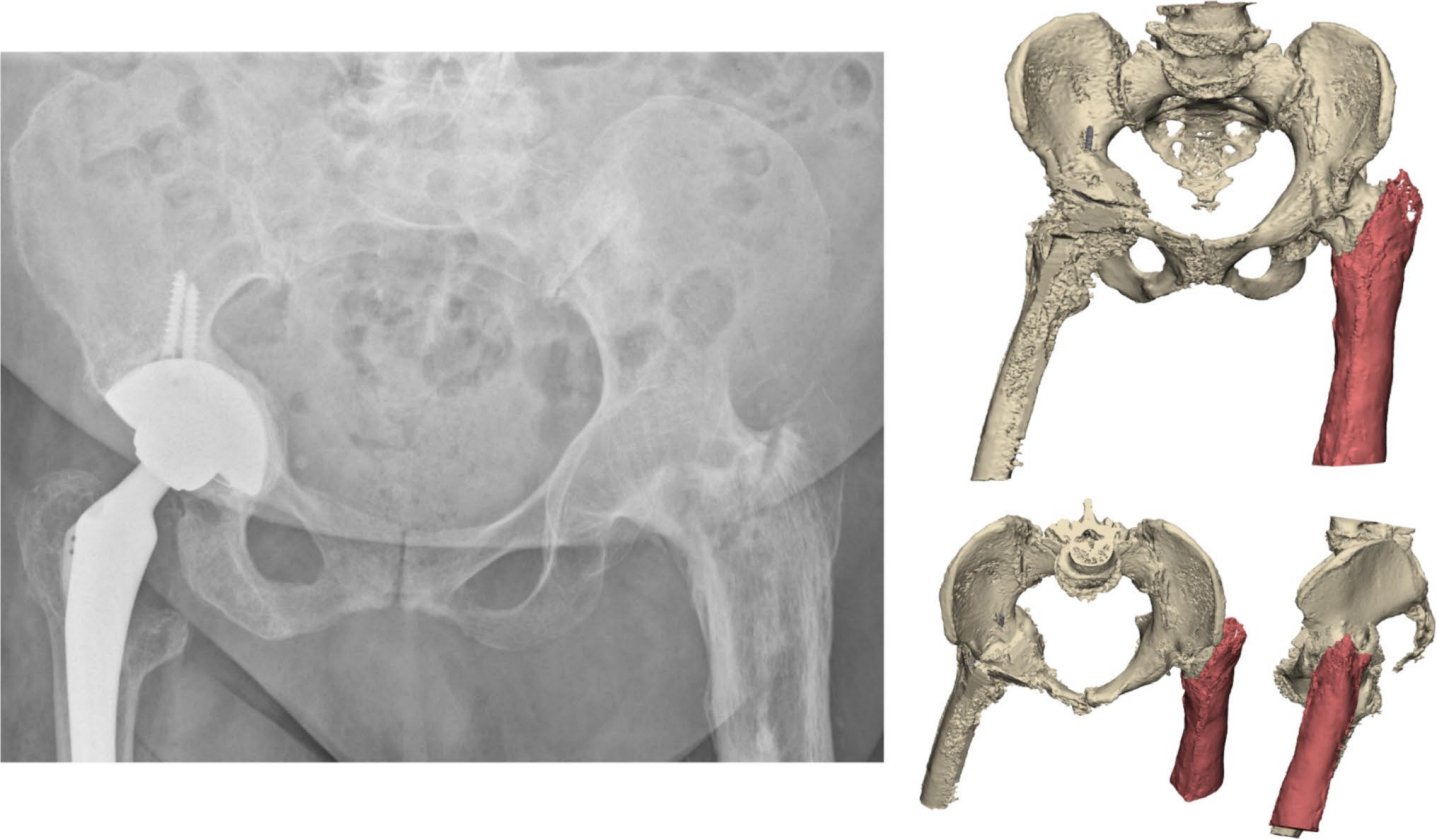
Case 3. Preoperative X-ray and 3D reconstruction of left intertrochanteric/lateral collum fracture in a an arthrodesed hip
